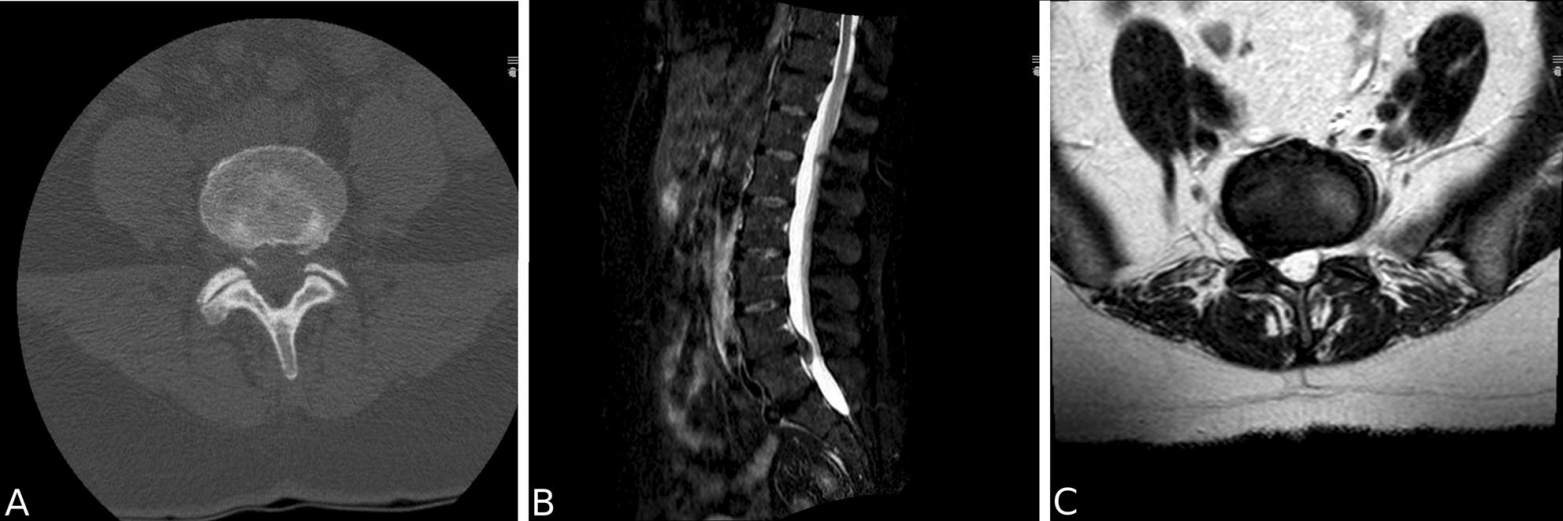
Sample CT and MR from one individual. (A) CT Axial with type 1b bone avulsion L4/L5. (B) STIR sagittal with disk extrusion L4/L5. (C) T2 axial with right disk extrusion L4/L5.
